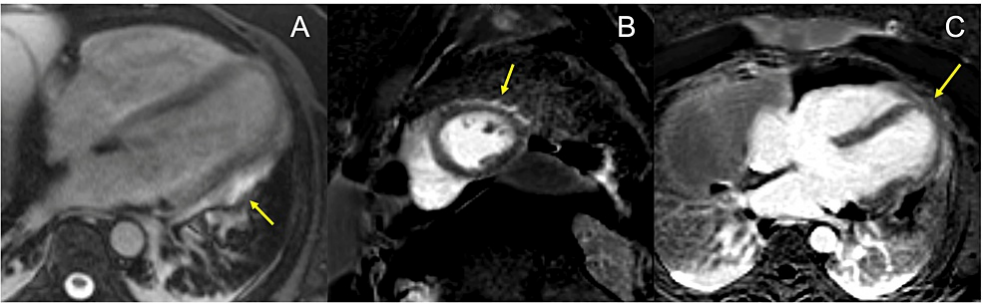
Cardiac MRI A. Cardiac MRI axial post-contrast True Fast Imaging with Steady-State Free Precession sequence: Thickening and enhancement of the pericardial edge (Yellow arrow) coinciding with morphological alteration site in the lateral wall of the left ventricle (Red arrow in A). B and C. Cardiac MRI Phase-Sensitive Inversion Recovery late enhancement sequence: No significant fibrosis at herniation site (Yellow arrow).
